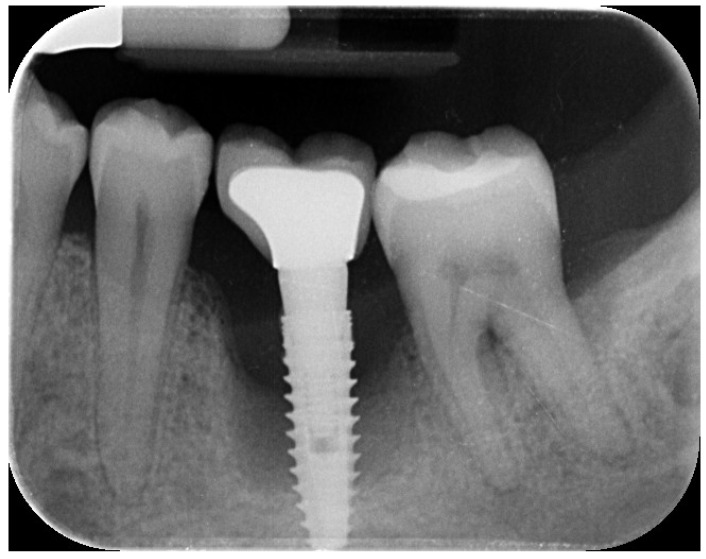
Initial radiographic assessment showing peri-implantitis on the implant and periodontal involvement on the second left mandibular molar.
